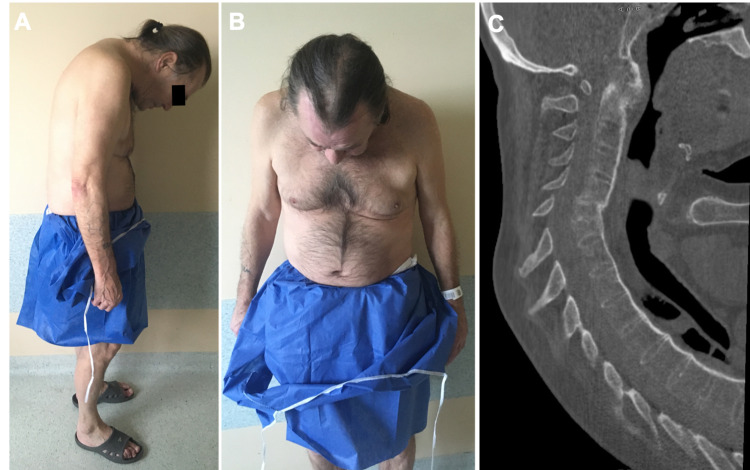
Patient with AS and the chin-on-chest deformity exacerbated by a C5 fracture A) and B) clinical images. B) CT sagittal MPR showing completely fused spine, displaced fracture, cervical hyperkyphosis AS: ankylosing spondylitis
